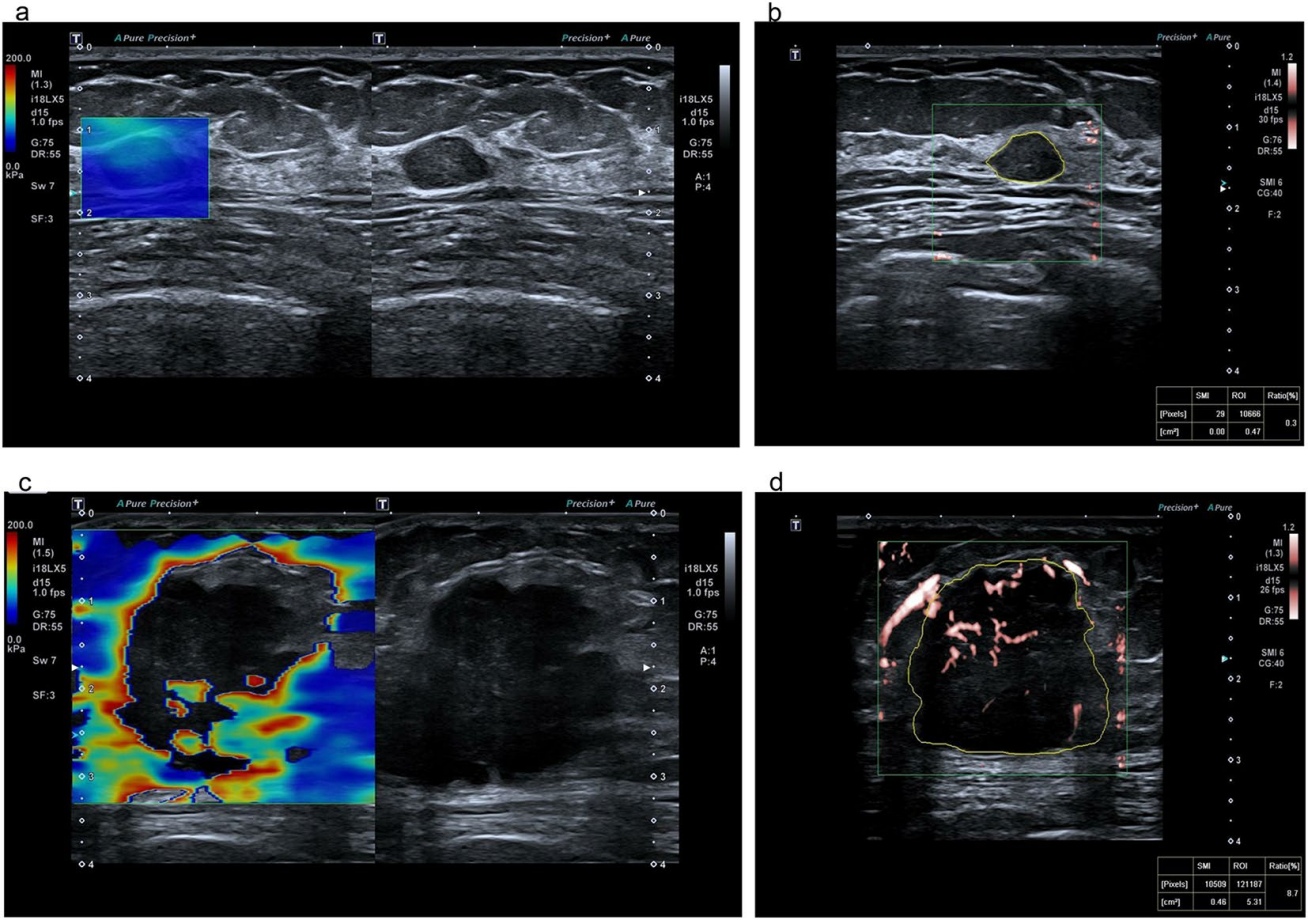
Examples of SWE and VI ultrasound images. A benign lesion with SWE, (a) 32.8 kPa and VI 0.3%, (b) and a malignant lesion with SWE, (c) 159.7 kPa and VI 8.7% (d).
